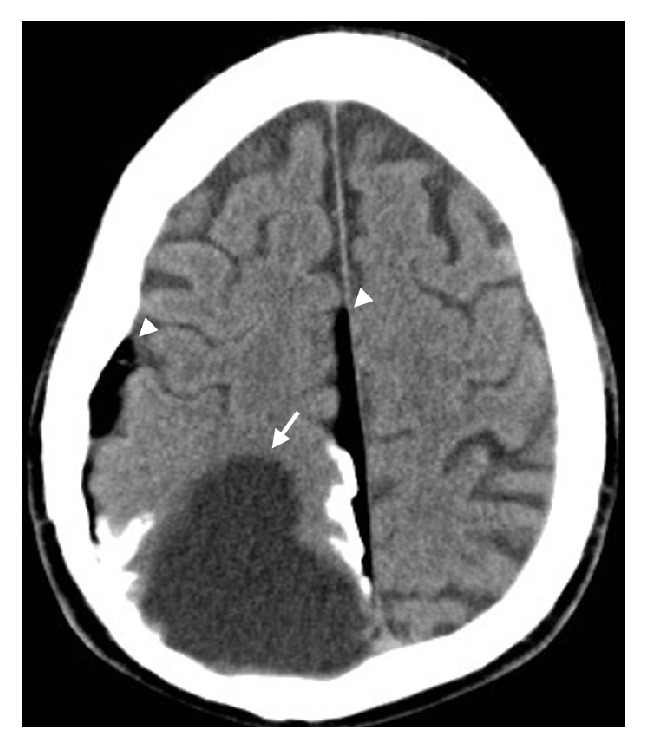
Noncontrast CT brain with a large right posterior parietal porencephalic cyst with adjacent cortical calcification (arrow). There is intracranial fat tracking along the falx and subjacent to the right parietal bone (arrowheads).
